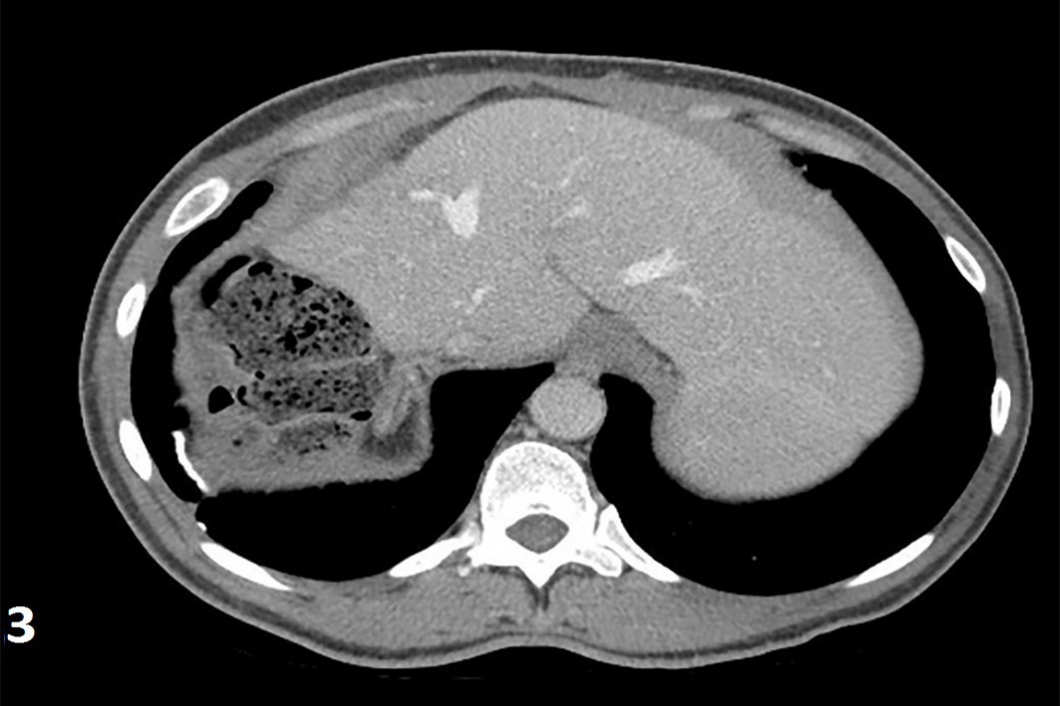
Computed tomography scan (abdomen) (after four weeks of antibiotics) showed complete resolving of the previously seen small collection/cyst anterior to the liver dome since the previous exam. No evidence of liver mass or collection. No evidence of perihepatic fluid or collection.
